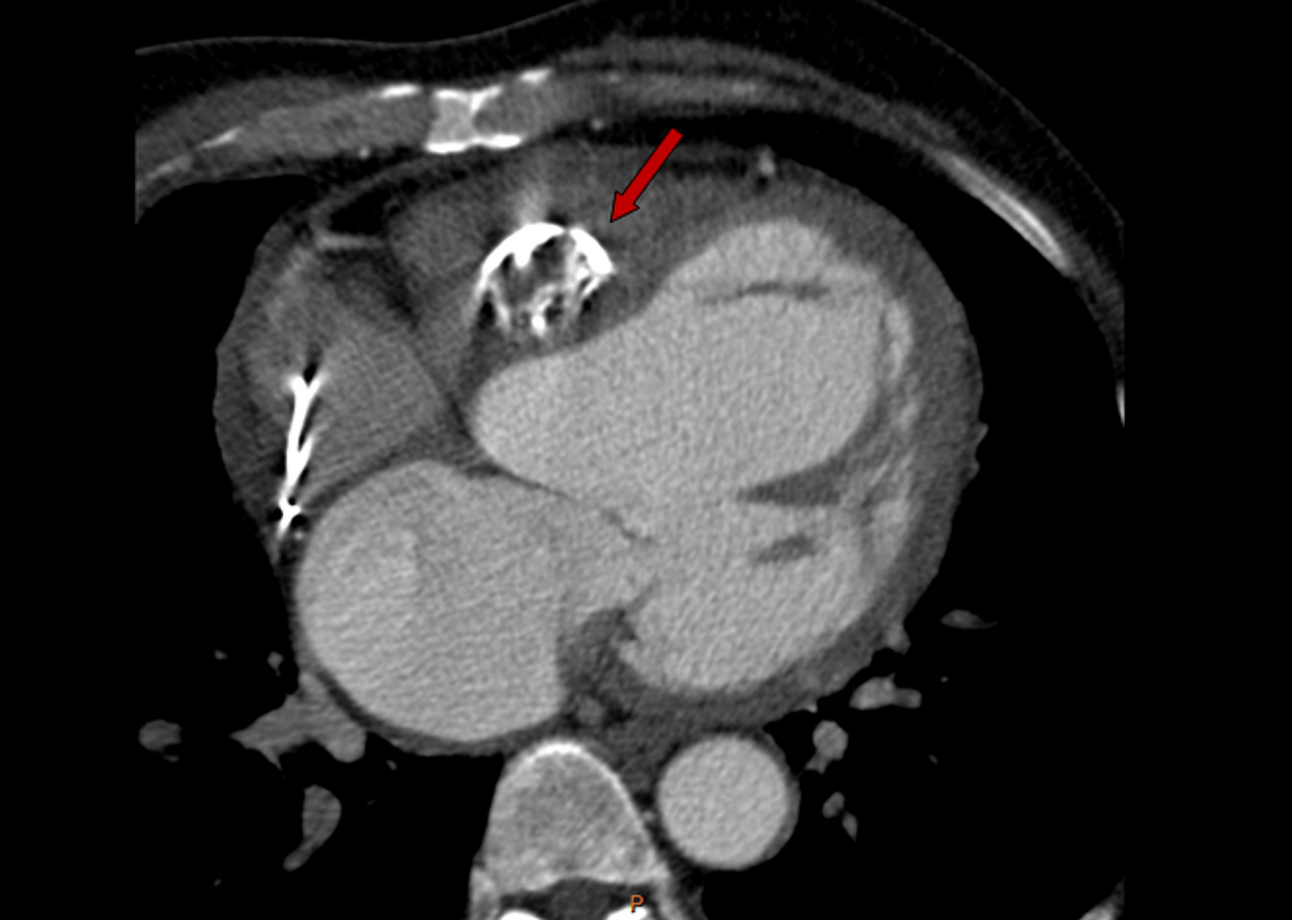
Computed tomography imaging showing a cyst located in basal interventricular septum with multiple calcifications
